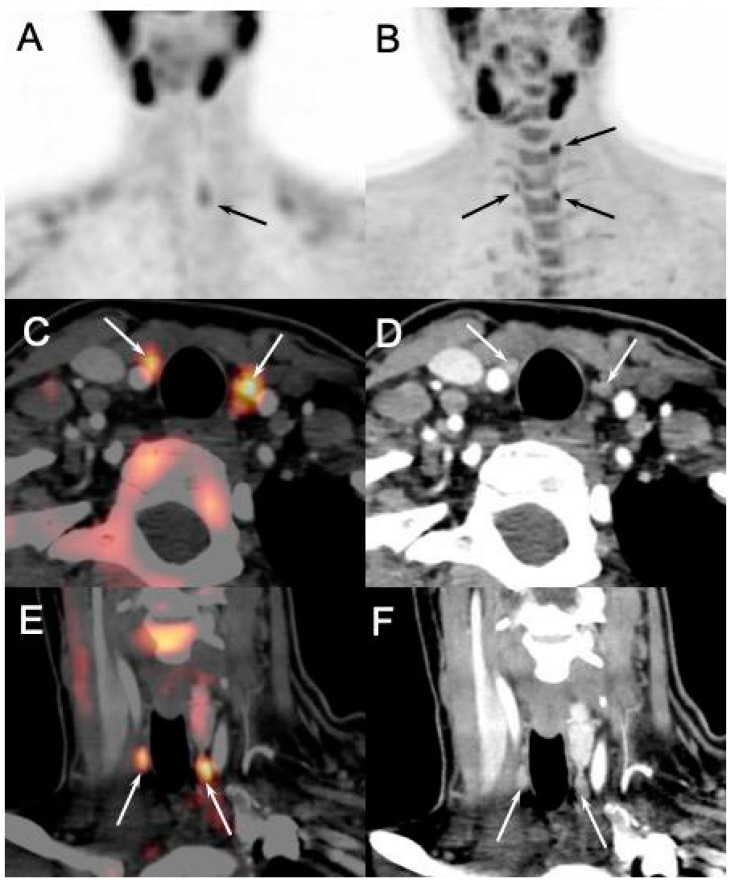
Discordant results of [^99m^Tc]sestamibi parathyroid scintigraphy ((A): anterior MIP) and [^18^F]FCH PET/4D-CT ((B): anterior MIP; (C,E): axial and coronal fusion images; (D,F): axial and coronal 4D-CT) performed in 28-y-old MEN1 patient with recurrent primary hyperparathyroidism (PTH: 133 ng/L; calcemia: 2.70 mmol/L). [^18^F]FCH PET/CT confirmed scintigraphy findings (pathological left inferior gland) and detected two more hyperfunctioning parathyroids tumors (arrows, inferior right and superior left) afterwards confirmed by pathology.
